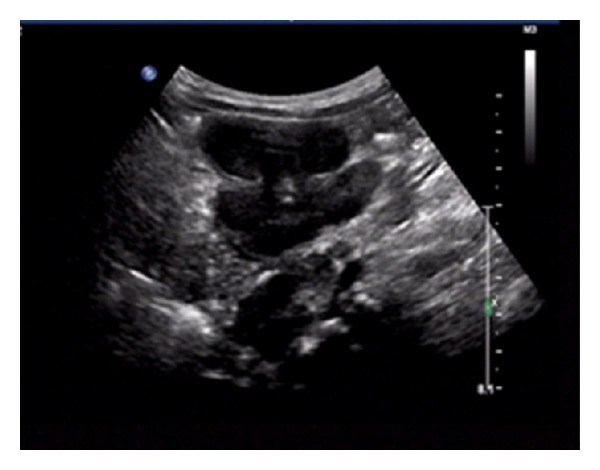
Ultrasound demonstrates multiple retroperitoneal lymph node enlargements.
